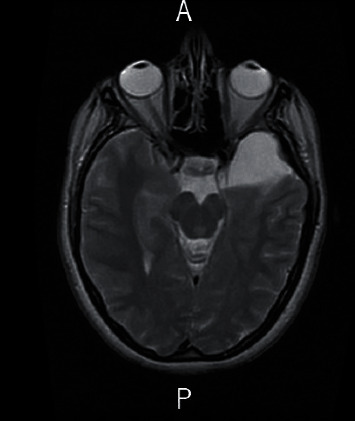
Axial T2-weighted view showing the big temporobasal arachnoid cyst communicating with the basal cisterns (Galassi II).
